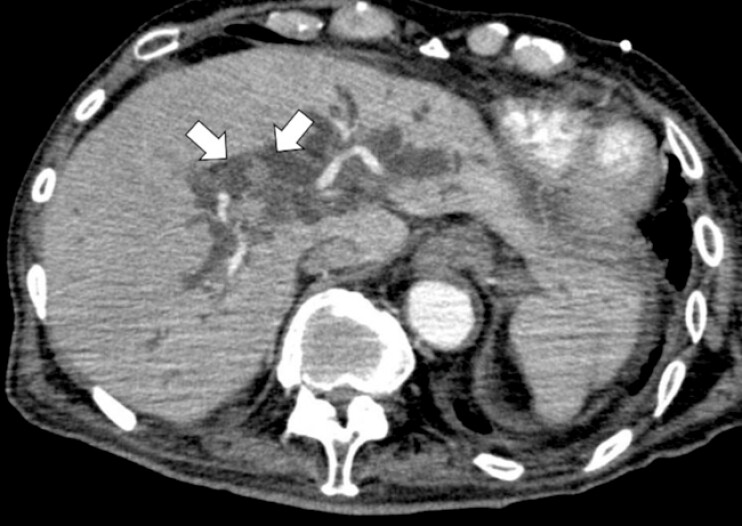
Contrast-enhanced CT showing a subtle lesion with faint enhancement at the orifice of the right anterior sectoral bile duct (arrow). CT, computed tomography.
